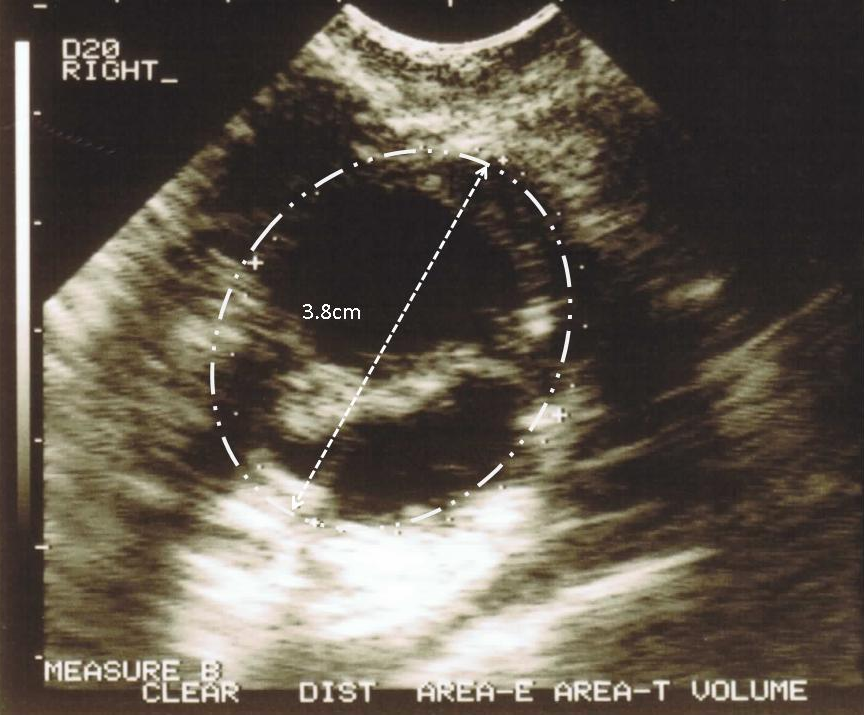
Transvaginal ultrasound image of septated right ovarian cyst in IVF, which reappeared after puncture performed prior to gonadotropin therapy. Aspirated fluid was consistent with borderline vs. well-differentiated ovarian serous adenocarcinoma.
